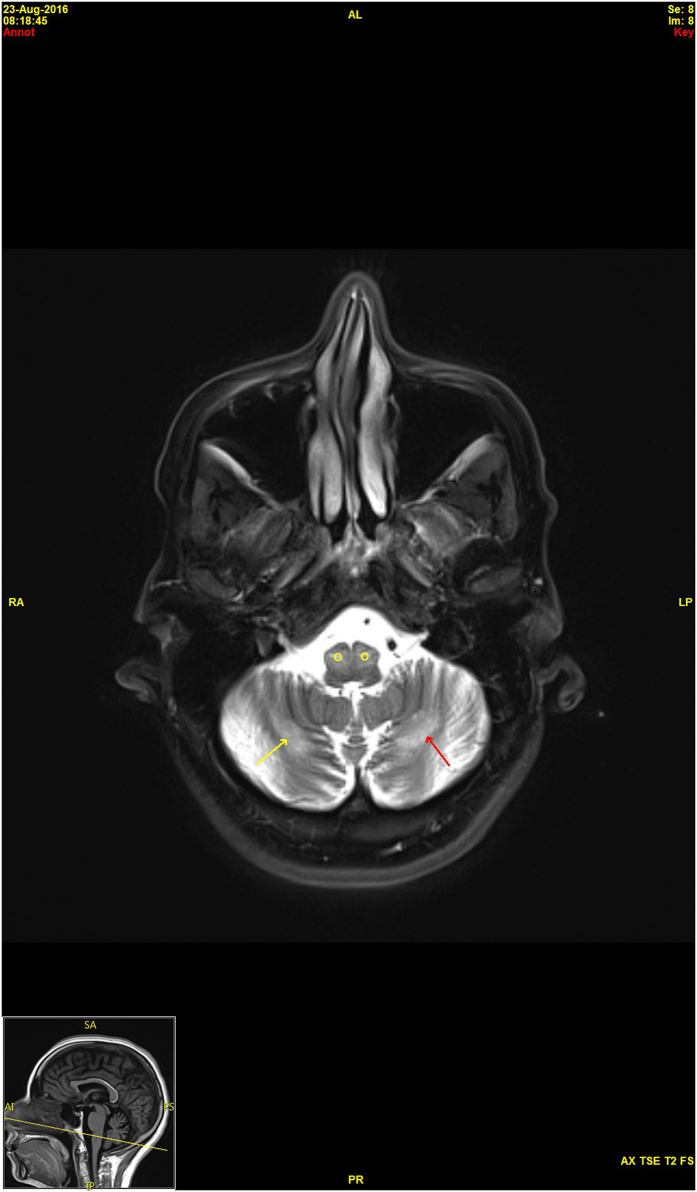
Axial T2 magnetic resonance imaging brain scan demonstrates bilateral, symmetrical hyperintense, hypertrophic olivary degeneration (O) and symmetrical bands of hyperintensity (arrows) in the cerebellar hemispheres.
